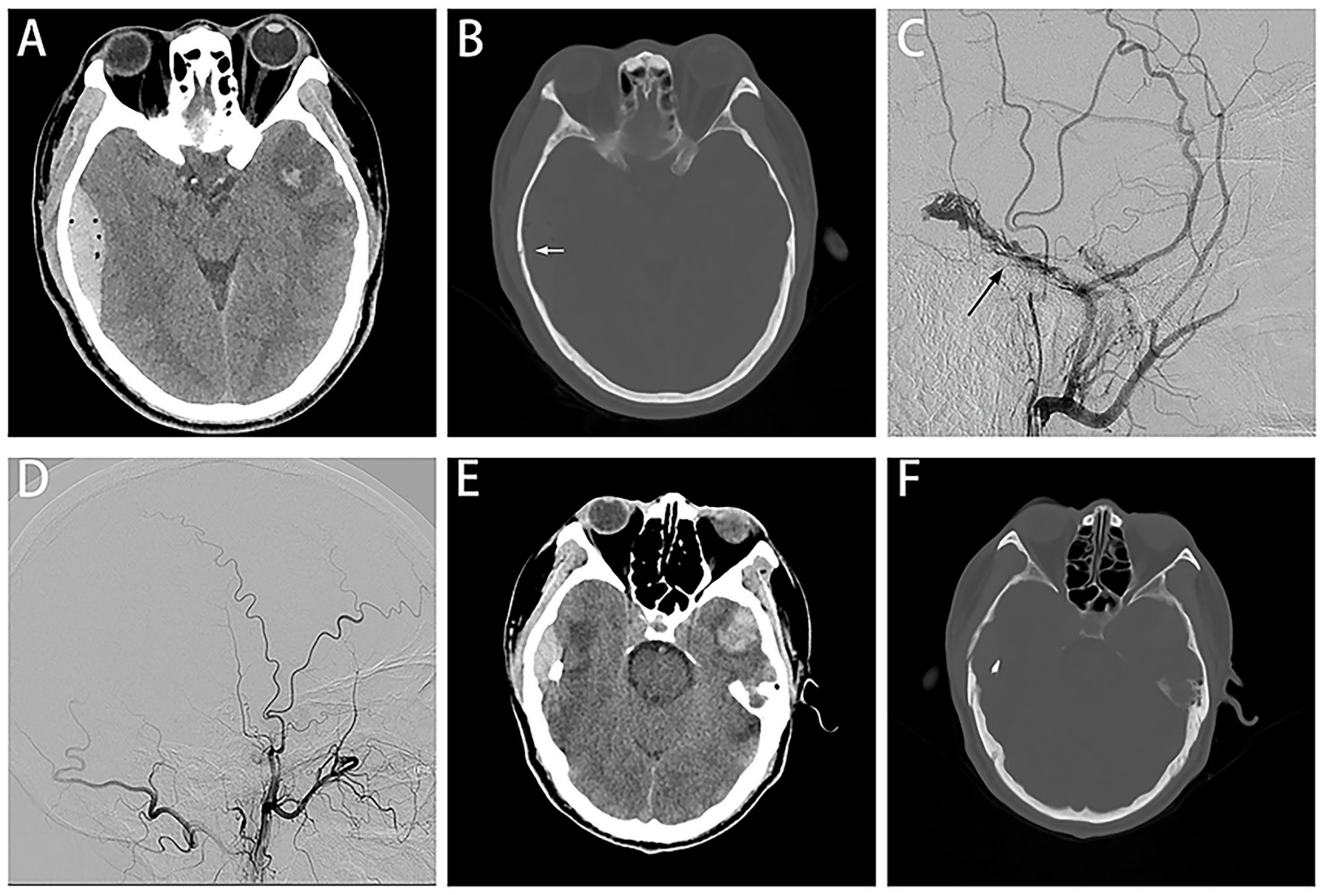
Case 1 representative images. CT scans show right temporal EDH with related fracture (A,B; white arrow), selective ECA angiography revealed MMA abnormally expanded and early venous drainage raising suspicion for traumatic DAVF (C), angiographic view after embolization (D), follow-up CT scan demonstrated no evidence of hematoma enlargement (E,F).
